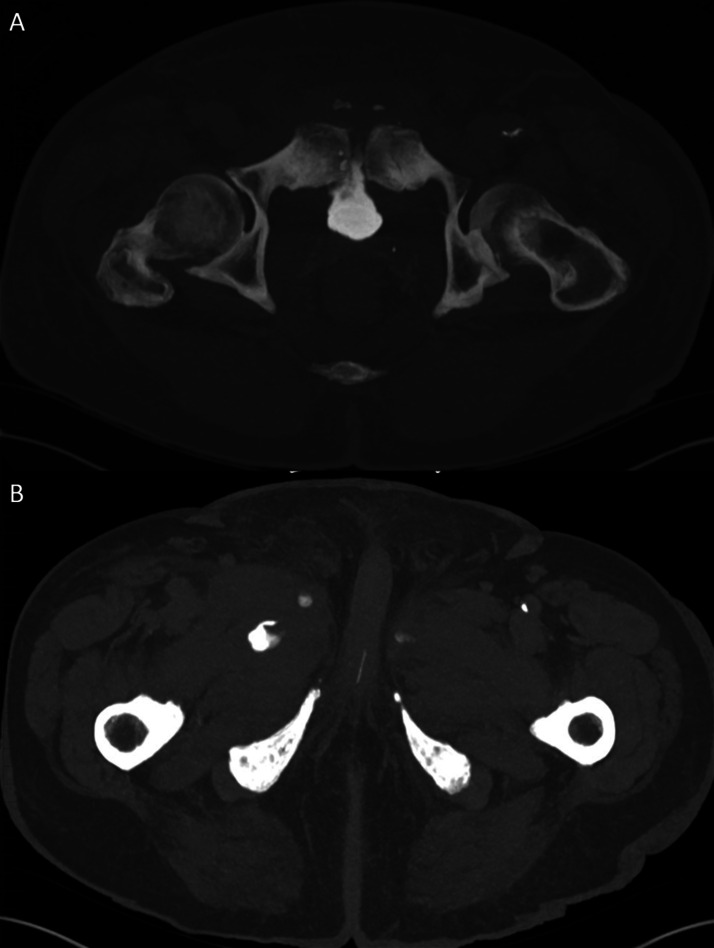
Delayed-phase CT demonstrating a direct fistulous tract between the bladder and pubic symphysis (A) and contrast leakage into the bilateral adductor compartments (B).
